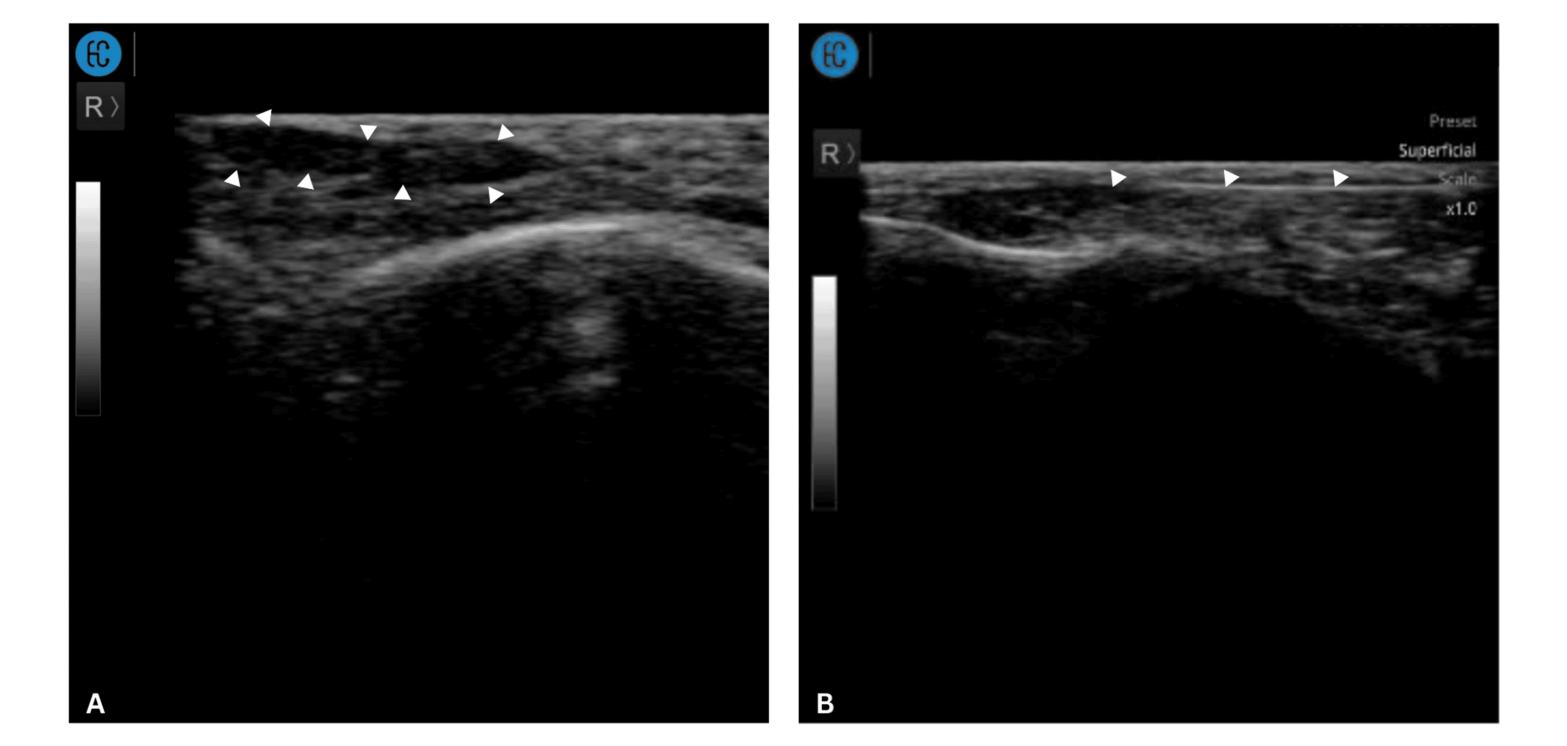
Ultrasonographic findings and ultrasound-guided treatment of the tear trough filler nodule (Case 1) (A) Ultrasound image of the left infraorbital region in the oblique plane demonstrating a well-defined hypoechoic lesion (indicated by arrowheads) within the superficial subcutaneous layer, located superficial to the orbicularis oculi muscle, consistent with aggregated filler material. (B) Ultrasound image obtained during ultrasound-guided intralesional injection of the filler nodule with arrowheads indicating the cannula.
